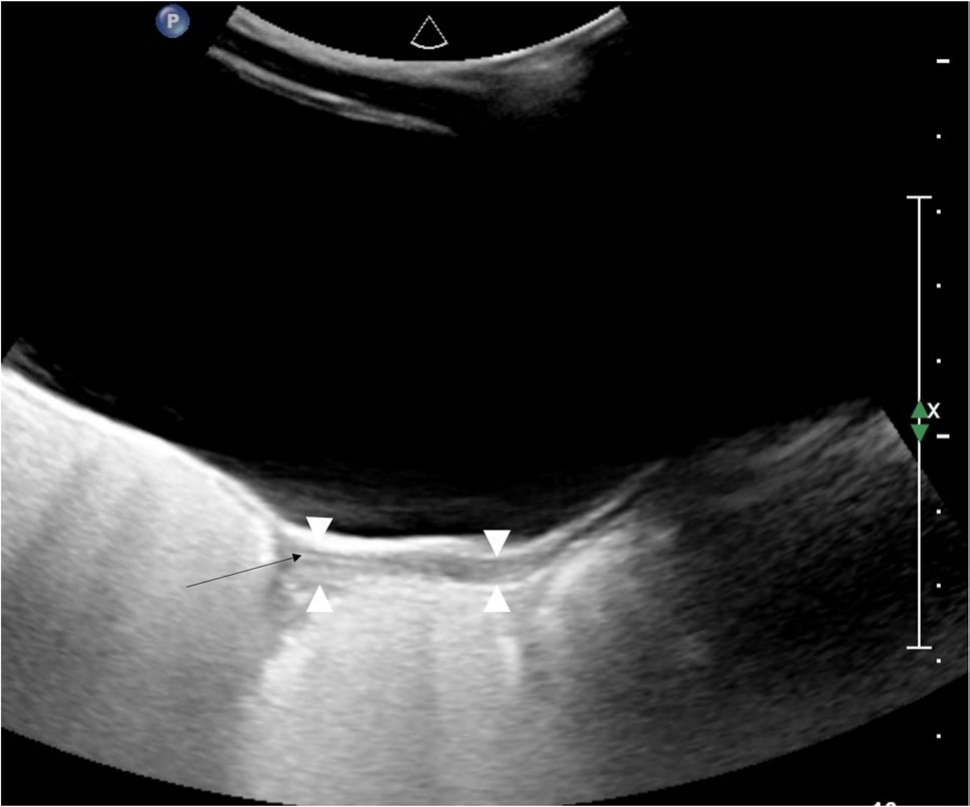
Prepubertal 8-year-old girl. Sagittal gray-scale pelvic ultrasound in an 8-year-old prepubertal girl shows a small tubular uterus in which the anteroposterior diameters of cervix and fundus (*arrowheads*) are equal*.* Cervix and fundus cannot be individualized. The endometrium is thin (*black arrow*)
